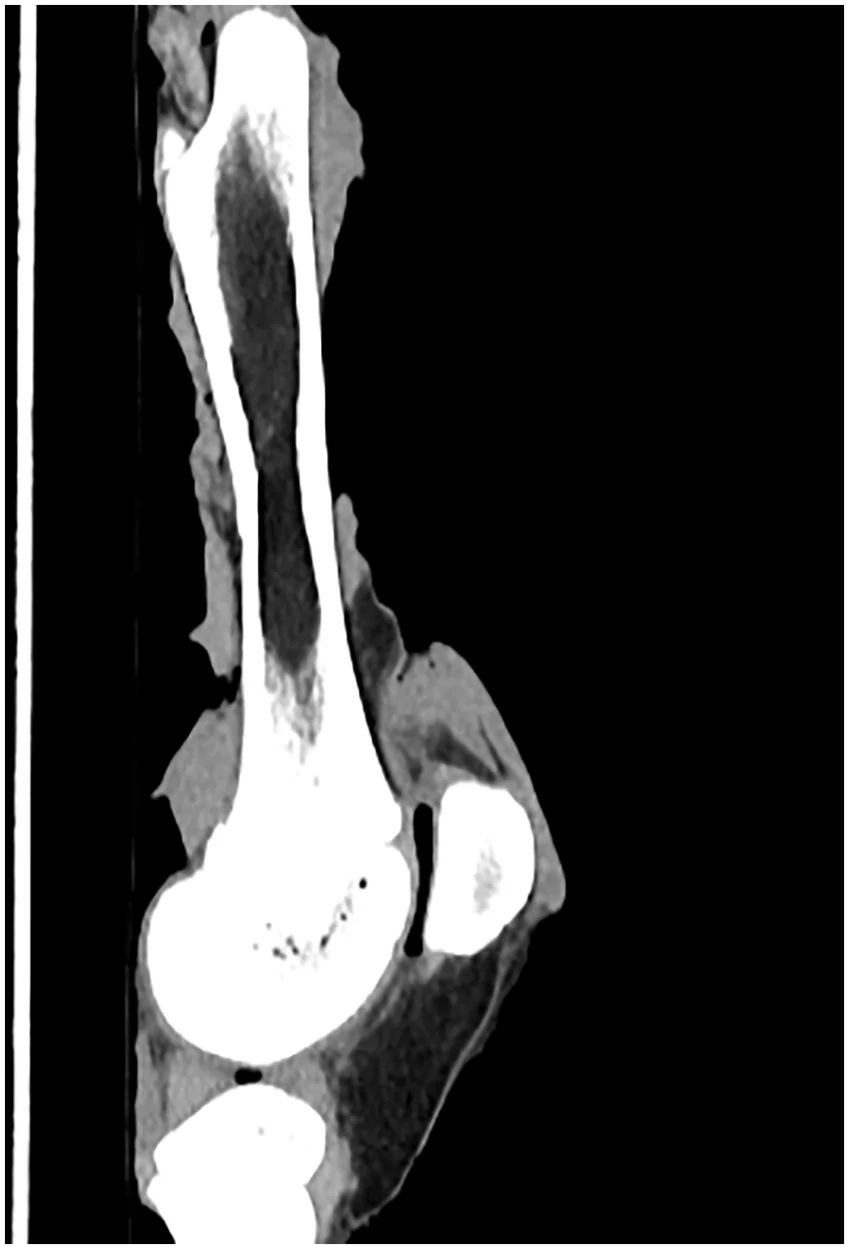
Standardized lateral-view correction before CT three-dimensional reconstruction. The posterior contours of the medial and lateral femoral condyles were overlapped to obtain a consistent measurement plane.
